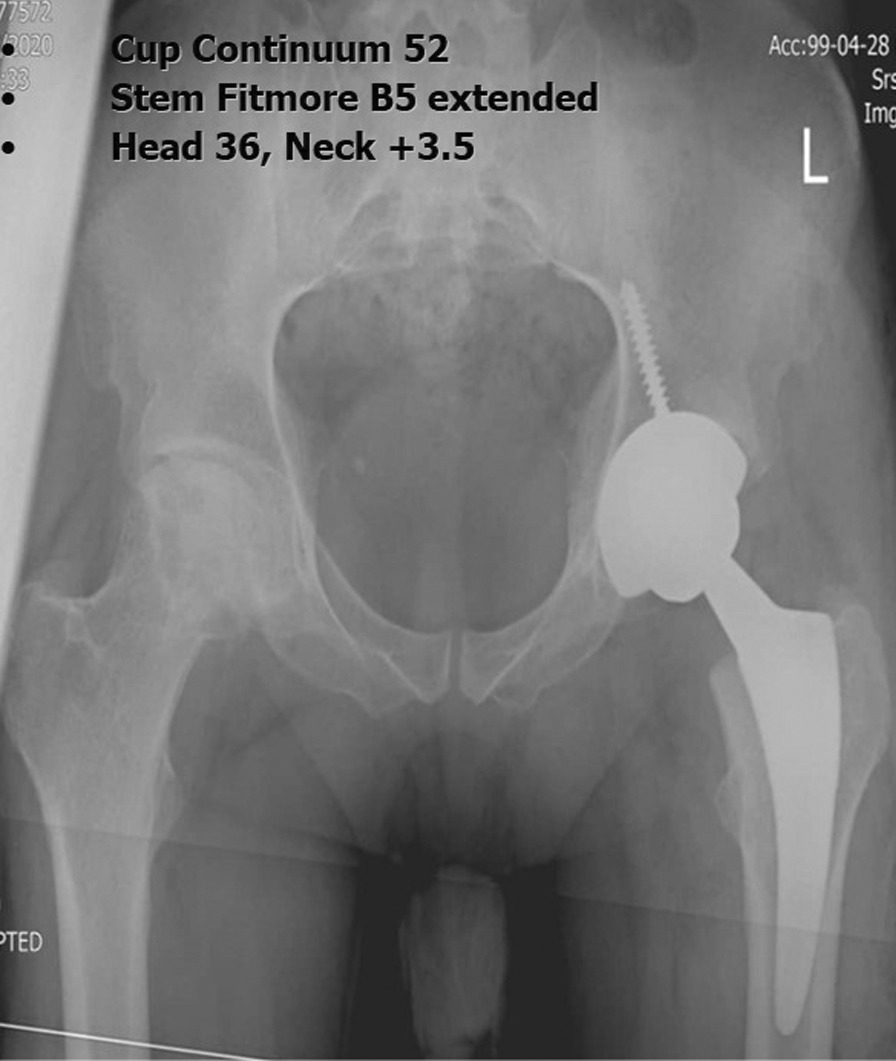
Postoperative radiograph and implant details
